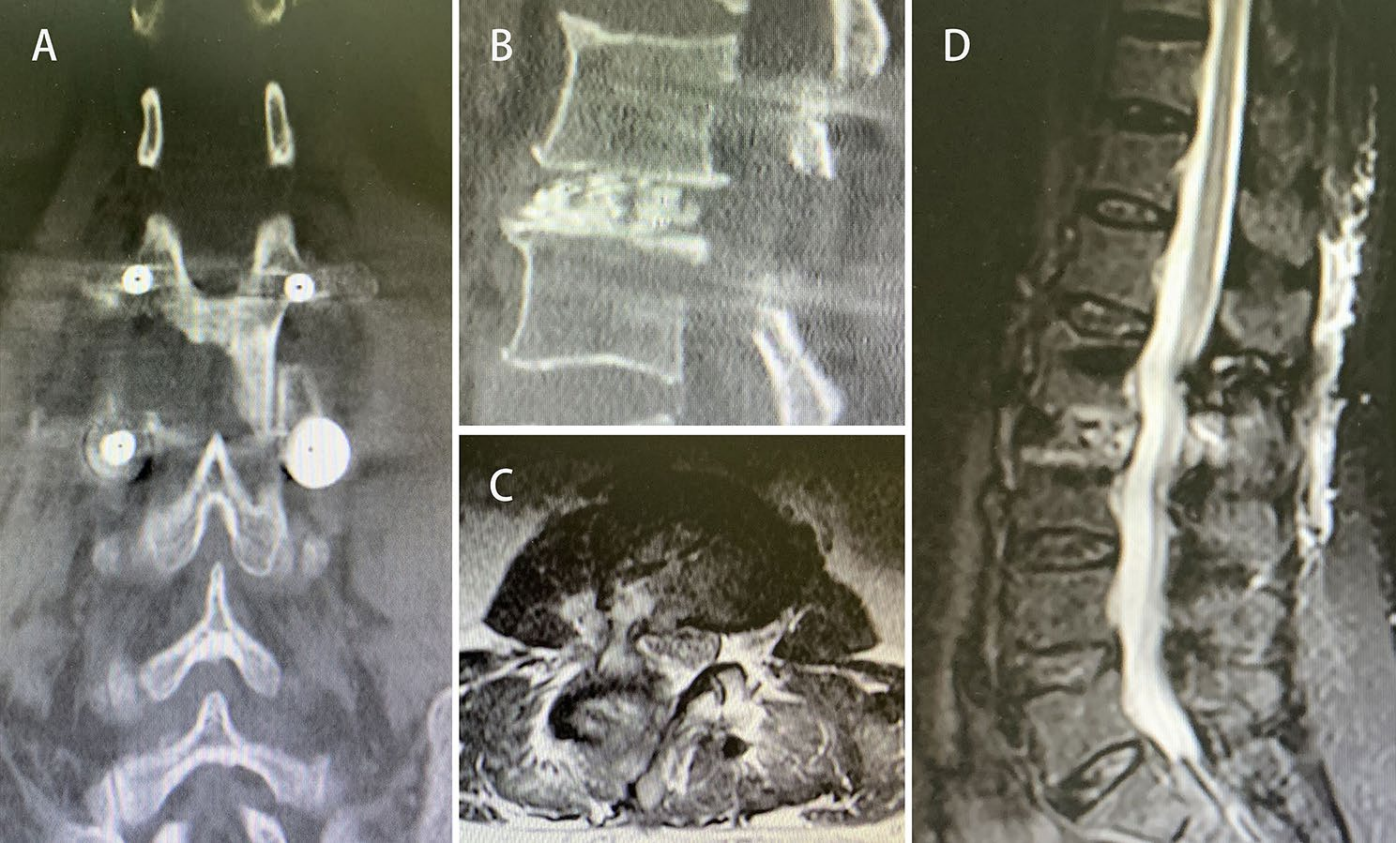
Postsurgical CT and MRI showing sufficient intervertebral bone grafting and complete decompression. (A) Extent of laminectomy in coronal CT. (B) Sufficient intervertebral bone grafting in sagittal CT. (C and D) Complete decompression in MRI images
